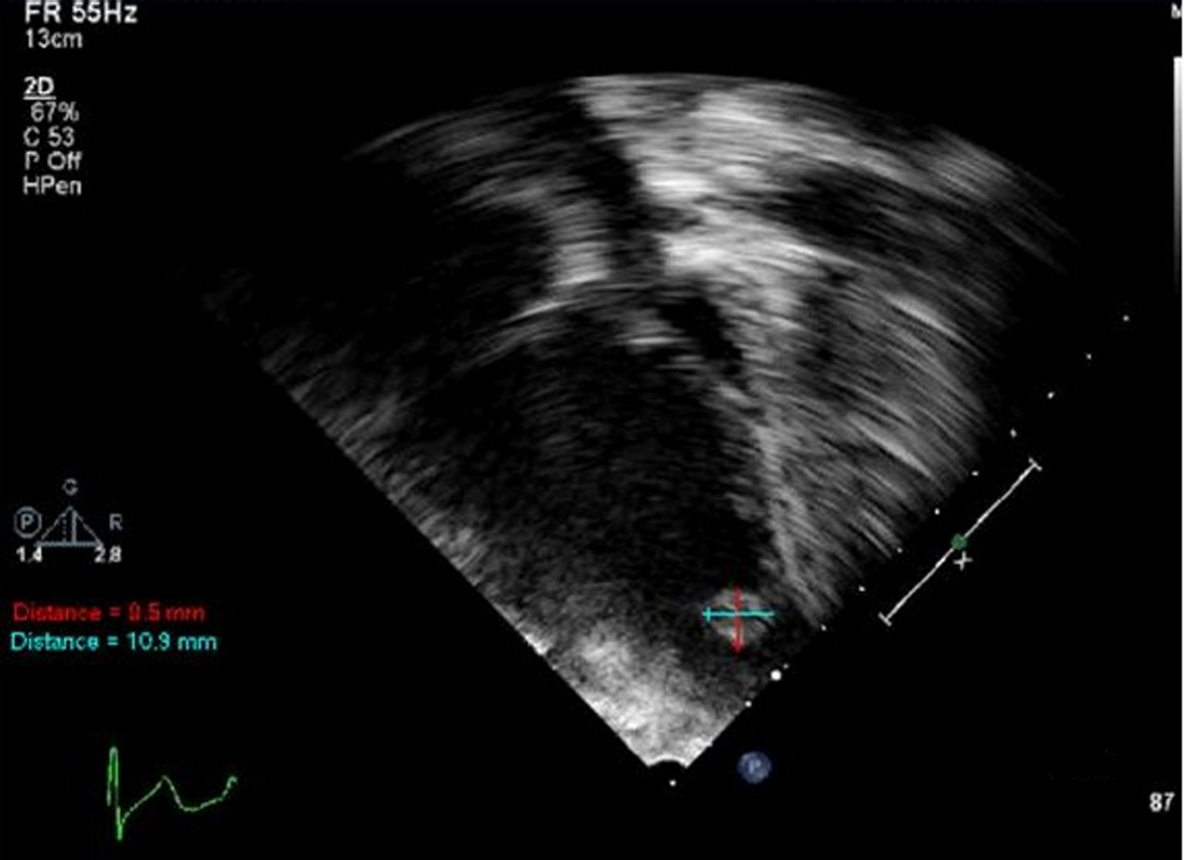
Transthoracic 2D echocardiogram. Apical four chamber view demonstrating a pedunculated mass near the apex of the left ventricle measuring 0.9 cm × 1.1 cm (arrows).
